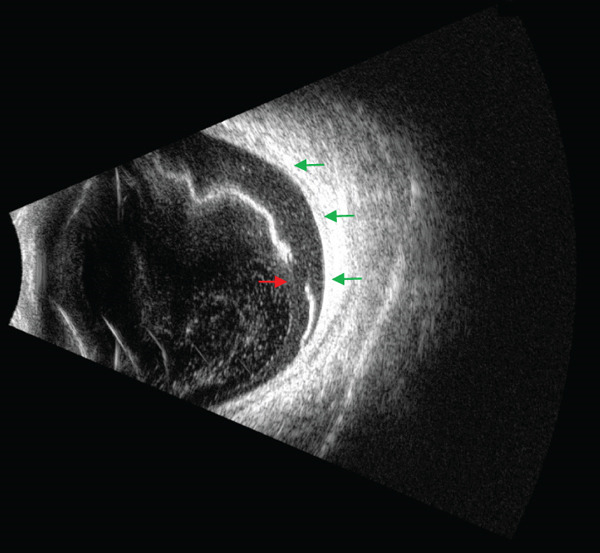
Preoperative ultrasound image of the rhegmatogenous retinal detachment, showing the retinal tear (red arrow) and a flattened choroid (green arrows) beneath the detachment, with no indications of malignancy or active tumor growth.
